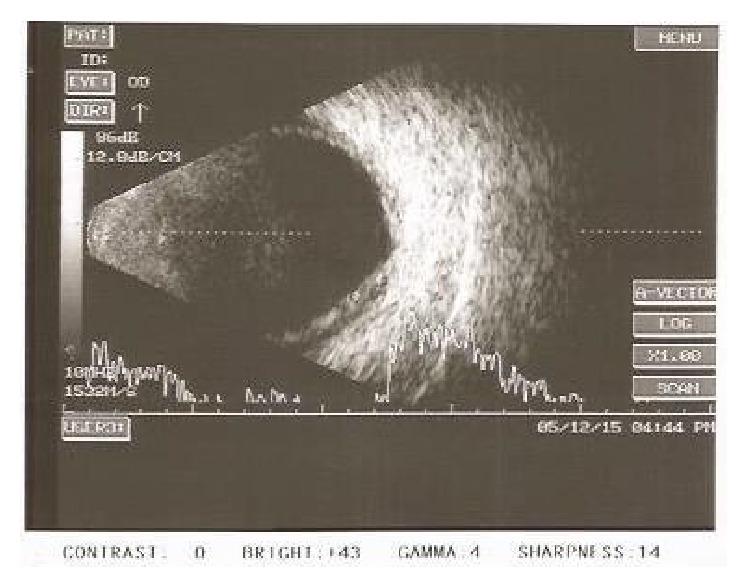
Right eye of the patient in Case Report 2: B-scan ultrasonography shows shallow choroidal detachment.
